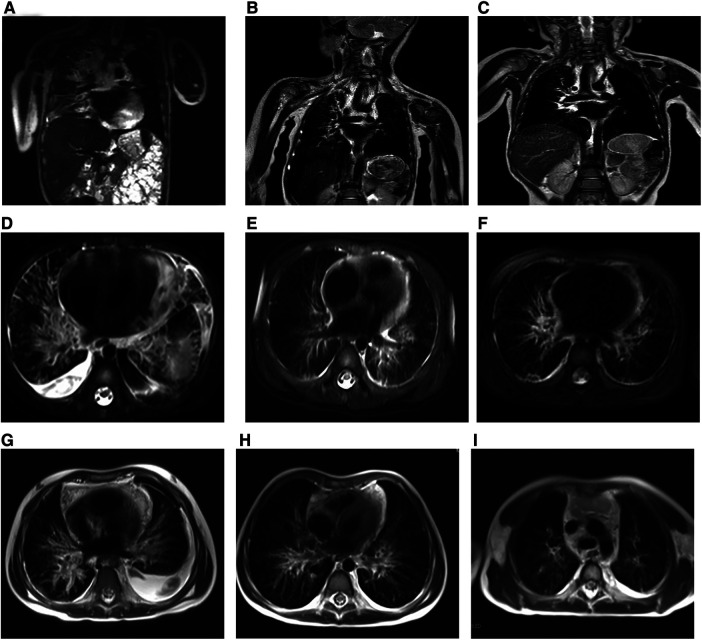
Preoperative and postoperative radiologic findings in KLA (Patients 1–3). (A–C) (Case 1) (A) Preoperative image. Coronal T2-weighted MRI of the chest revealed that 6 (B) and 12 months (C) after surgery, the inflammation of the patient improved, and the pericardial effusion decreased significantly. (D–F) (Case 2) Preoperative image (D) and postoperative images at 6 (E) and 12 months (F). The inflammation improved, and the fluid accumulation decreased compared with the previous period. All images are horizontal T2-weighted MRI images. (G–I) (Case 3) The presented images are all horizontal T2-weighted MRI images. The child had a large amount of pleural effusion and significant pulmonary inflammation before surgery (G). The symptoms of the child improved gradually, and the pleural effusion had mostly disappeared by 6 months (H) after the operation. The inflammation improved 12 months after surgery (I).
